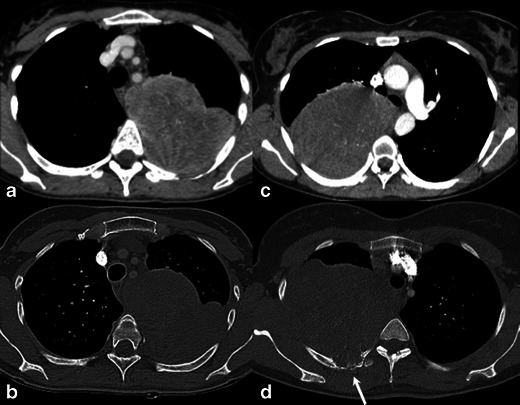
CT images of intrathoracic sarcomatous masses originating from chest wall. A 31-year-old woman (a and b) with Ewing sarcoma, a large mass with irregular shape, lobulated margins, heterogeneous enhancement, intratumoural vessels but no rib erosion. A 34-year-old woman (c and d) with a PNET, presenting an irregular mass, with smooth margins, heterogenous enhancement, rib erosion and intratumoural vessels—these showing a radial pattern in relation to the osseous destruction (*arrow* in d)
